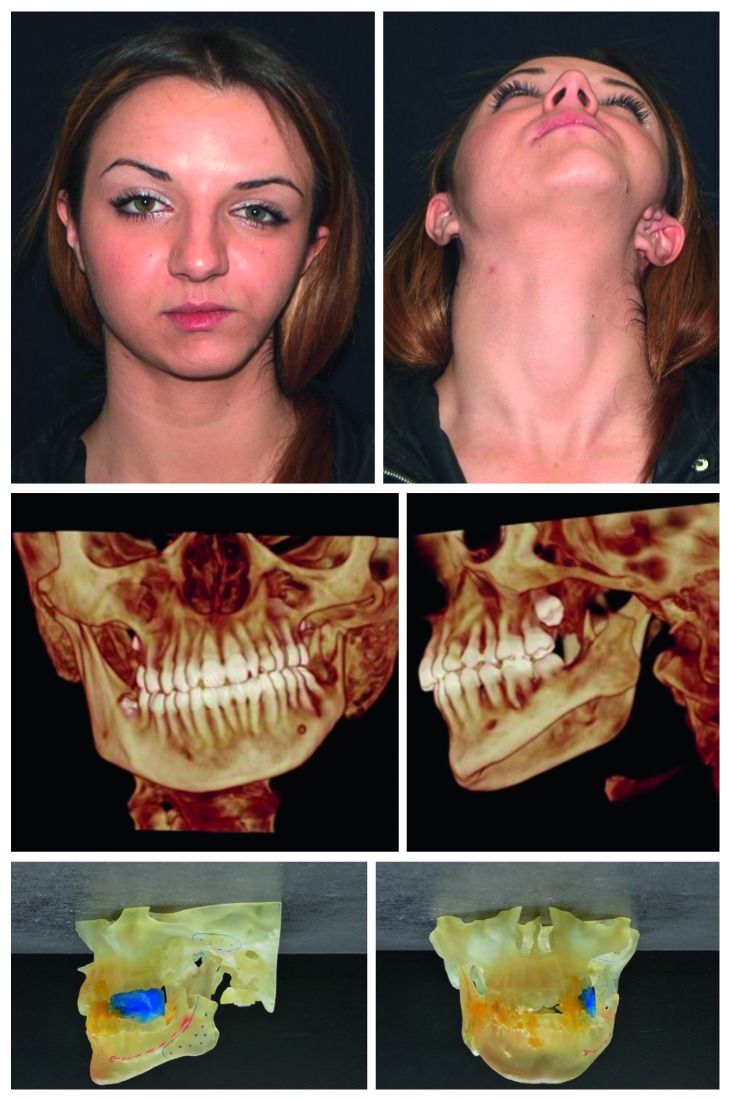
Patient presurgery in frontal view and axial view. 3D CT scan pre surgery in frontal and left lateral views. 3-dimensional plastic model of the patient's jaws, TMJs, and cranial base structures repositioned and fixed with quick-cure acrylic.
